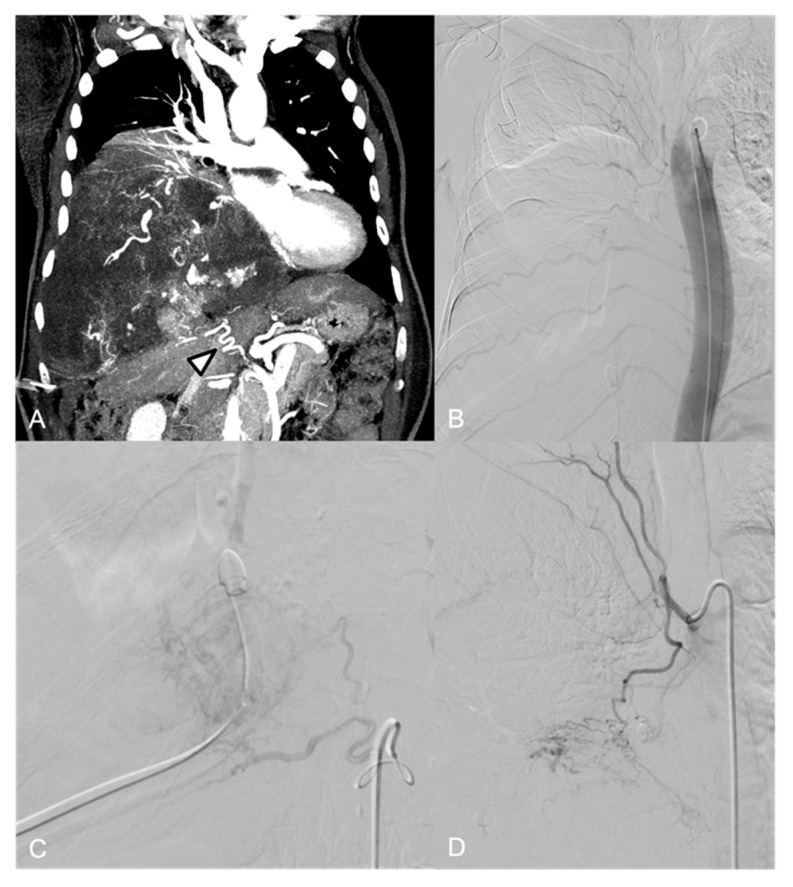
(A) CT Angiography, coronal view: large mass in the posterior right hemithorax. Vascular supply from the right phrenic artery arising from the abdominal aorta (arrowhead). (B) Preliminary aortography shows hypertrophy of right intercostal arteries. (C) Selective angiography of the right phrenic artery and (D) right intercostobronchial trunk, with their supply to the tumor.
